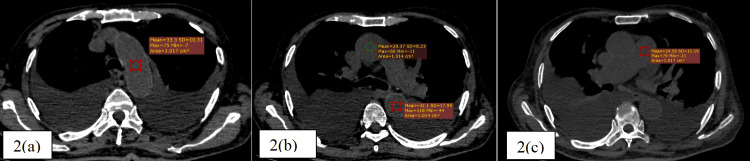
Non-contrast CT thorax-mediastinal window of a 74-year-old severe anemic male patient (serum hemoglobin 7.2 g/dl) showing the circular regions of interest (ROIs) in standardized anatomical sites, the ascending and descending thoracic aorta (a), aortic arch (b), and main pulmonary artery (c) with mean HU as +29.3, +32.1, +33.3, and +24.5, respectively
